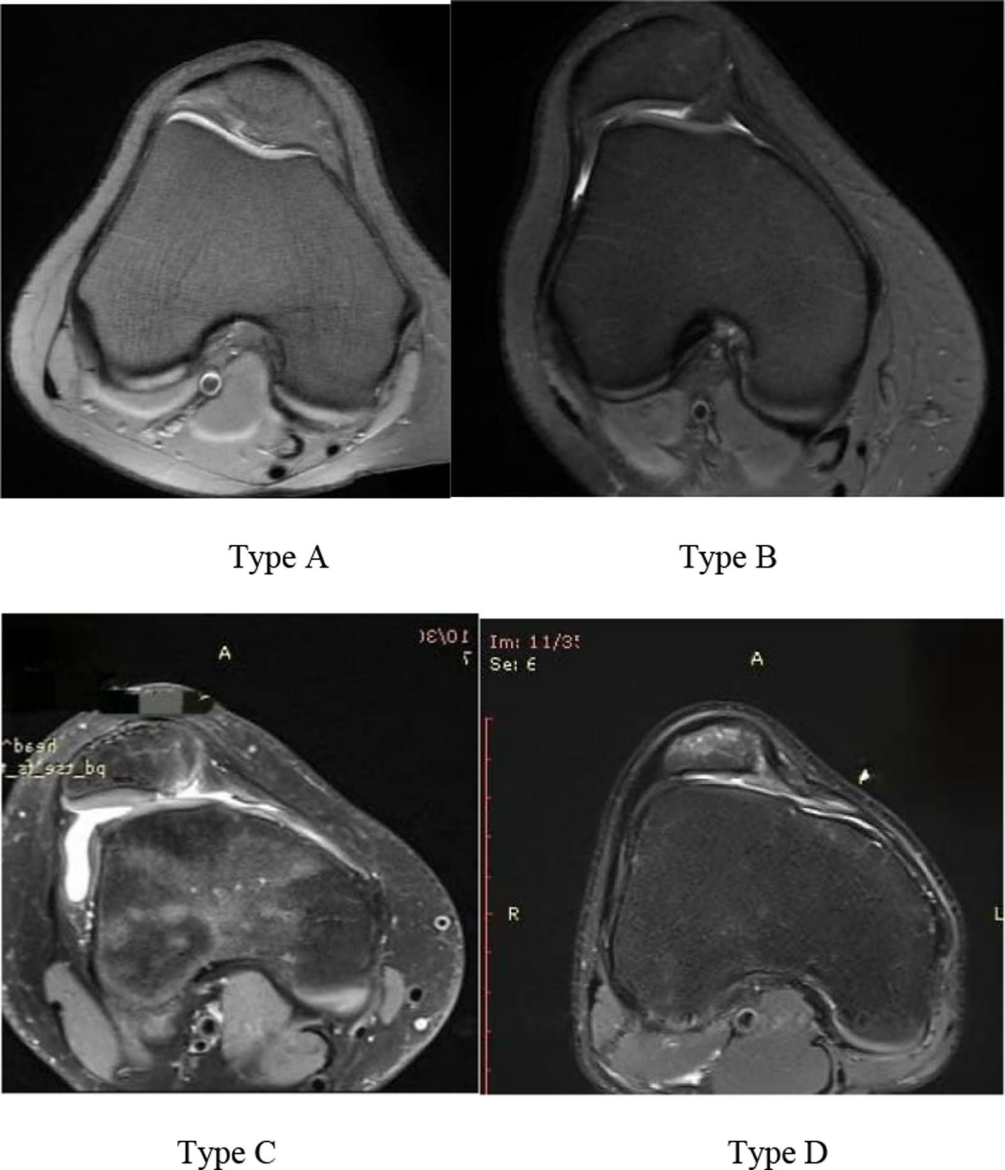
Various types of trochlear dysplasia shown on axial MRI
